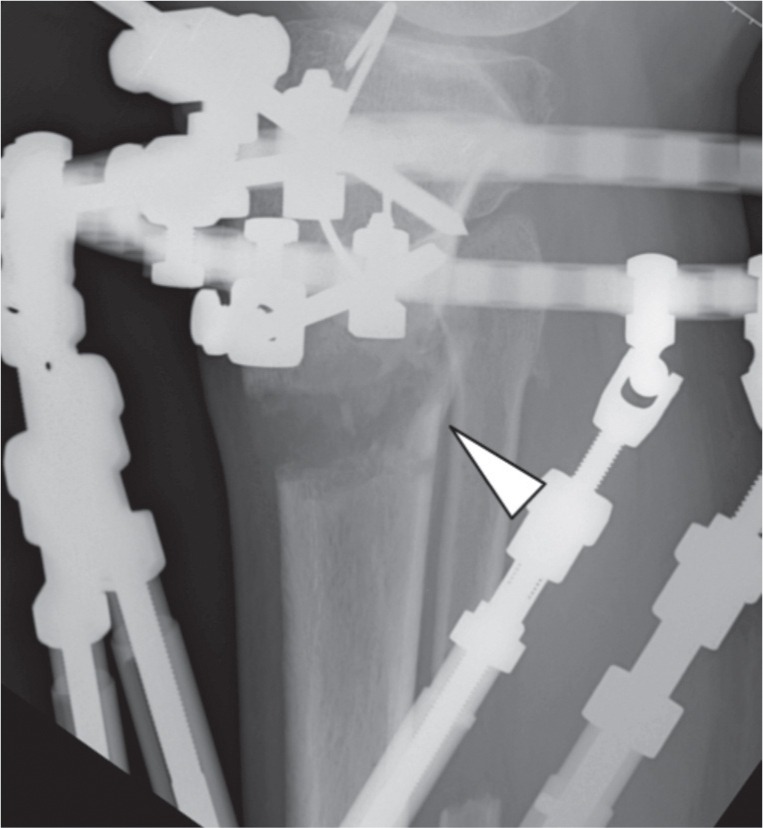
Radiograph showing Type II corticotomy with a “winglet”.
